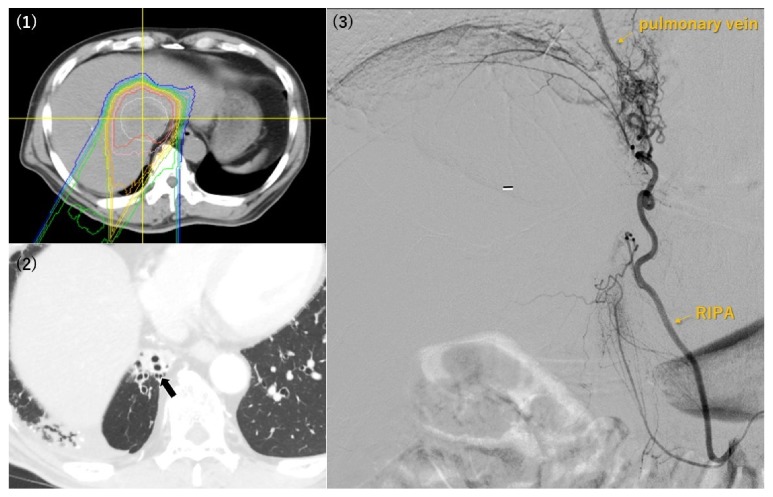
A 66-year-old man underwent his first proton beam therapy (PBT) treatment for hepatocellular carcinoma (HCC) in S8/1 48 months before the angiography procedure (1). Computed tomography revealed radiation-induced pneumonitis in the medial side of the right lower lung (2). An extrahepatic collateral pathway (via right inferior phrenic artery, RIPA) and an arteriovenous shunt for the pulmonary vein were noted during the procedure (3). The location of the arteriovenous shunt corresponded to the site of the radiation-induced pneumonitis.
